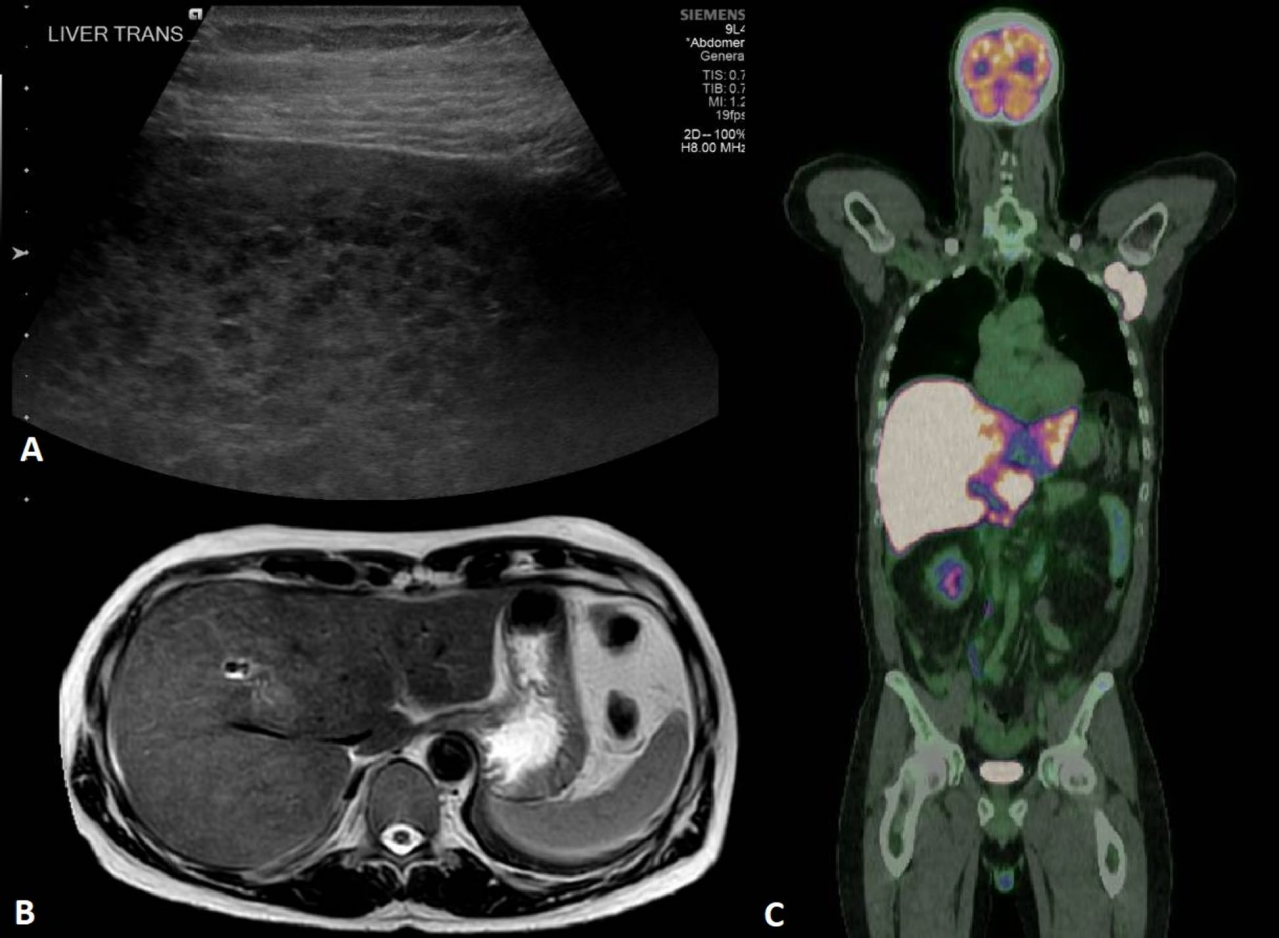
A Abdominal ultrasound demonstrating hepatic nodularity; B MRCP demonstrating a diffusely abnormal and heterogenous liver; C FDG-PET/CT scan revealed intensely FDG avid left axillary, peripancreatic and portocaval lymphadenopathy
